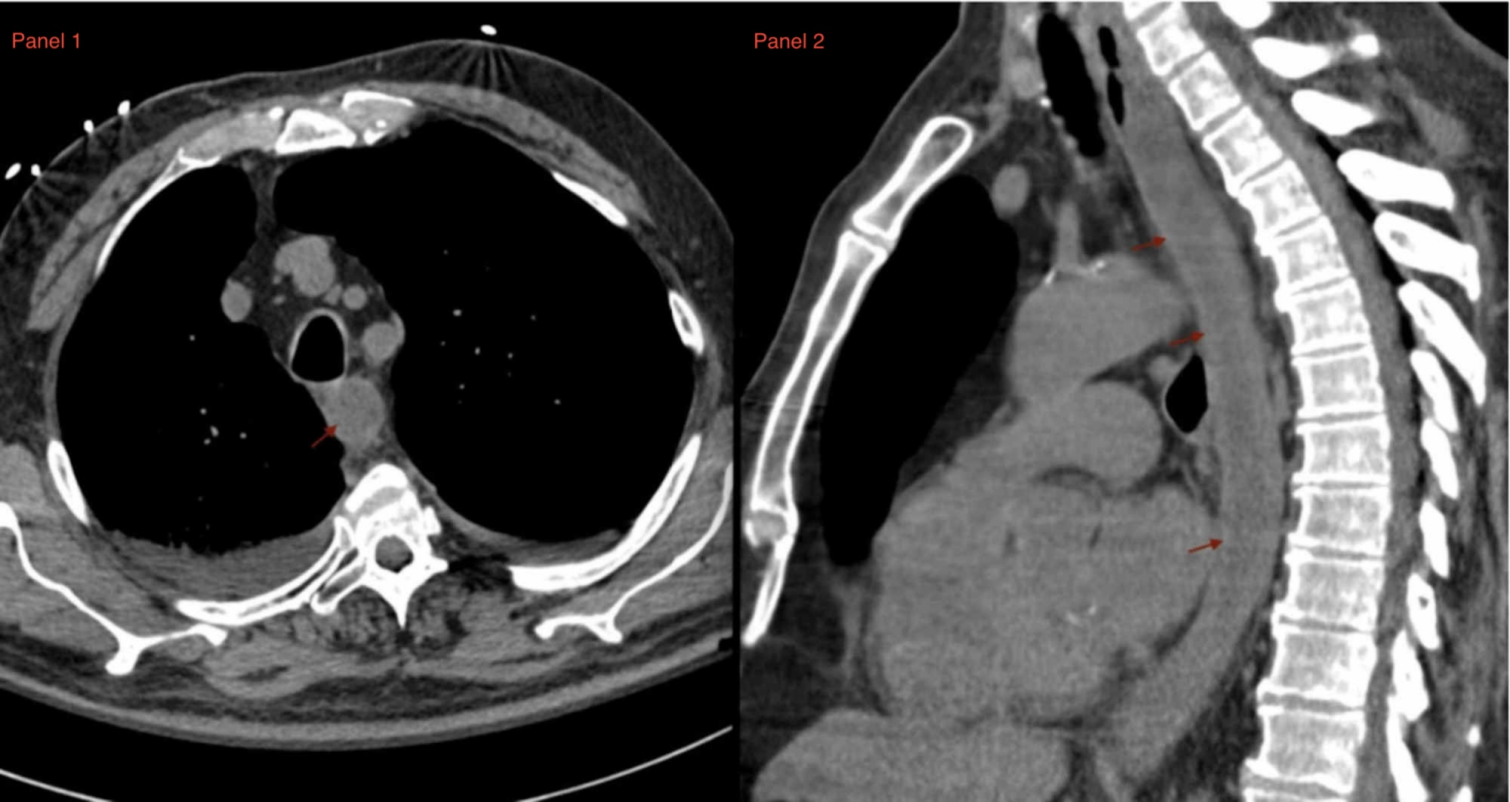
Panel 1: axial CT of the chest showing distal esophageal thickening. Panel 2: sagittal CT of the chest demonstrating distal esophageal thickening
